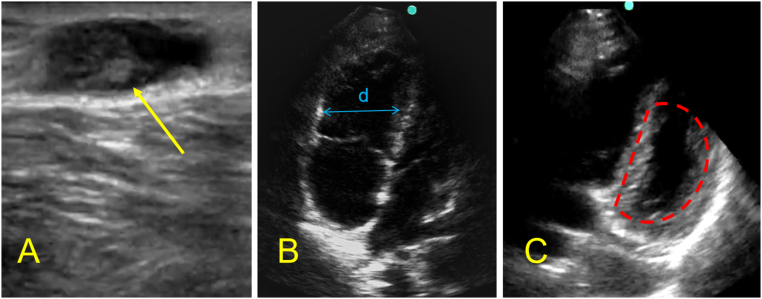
The lower extremity and cardiac point-of-care ultrasound of patient 2 after thrombolytic therapy. A: right great saphenous vein visible thrombosis (yellow arrow); B: apical four-chamber window shows significant enlargement of right ventricular (blue double arrow), RV: LV > 1, C: Parasternal short axis shows D shape sign (red dash line).
